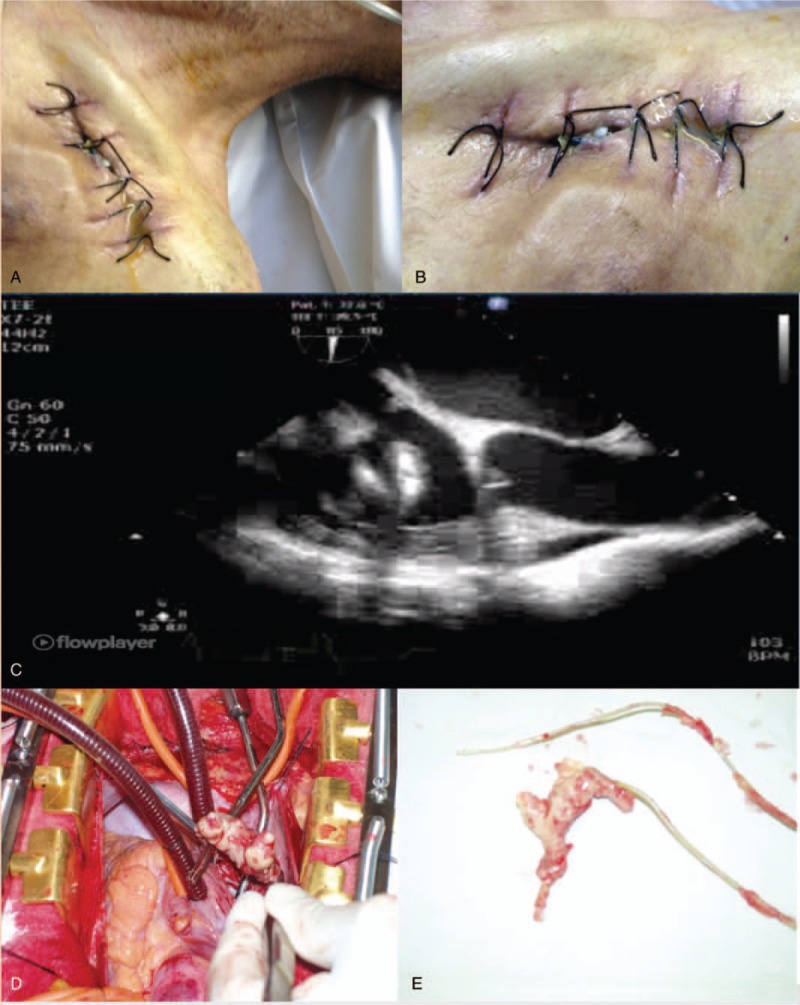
Skin changes seen in patient presenting with *Enterobacter cloacae* cardiac implantable electronic device infection (Panels A and B), septic shock and a large vegetation on transesophageal echocardiography (Panel C) on the lead that was surgically removed (Panels D and E).
